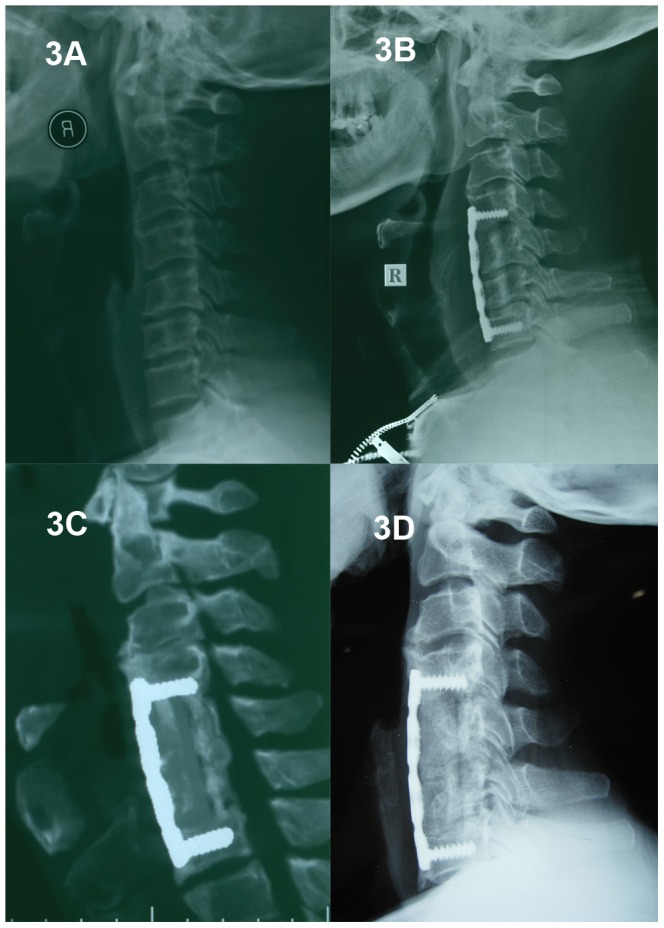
A 61-year-old male who underwent 2-level corpectomy with a nano-hydroxyapatite/polyamide66 cage used for cervical reconstruction. A preoperative cervical X-ray film (3A) shows a loss of cervical lordosis. The immediately postoperative lateral X-ray (3B) shows C5 and C6 corpectomy and the n-HA/PA66 cage used for reconstruction. The 3D-CT (3C) and lateral X-ray (3D) show obvious bony fusion and restoration of cervical alignment at the final follow-up (four years and four months).
